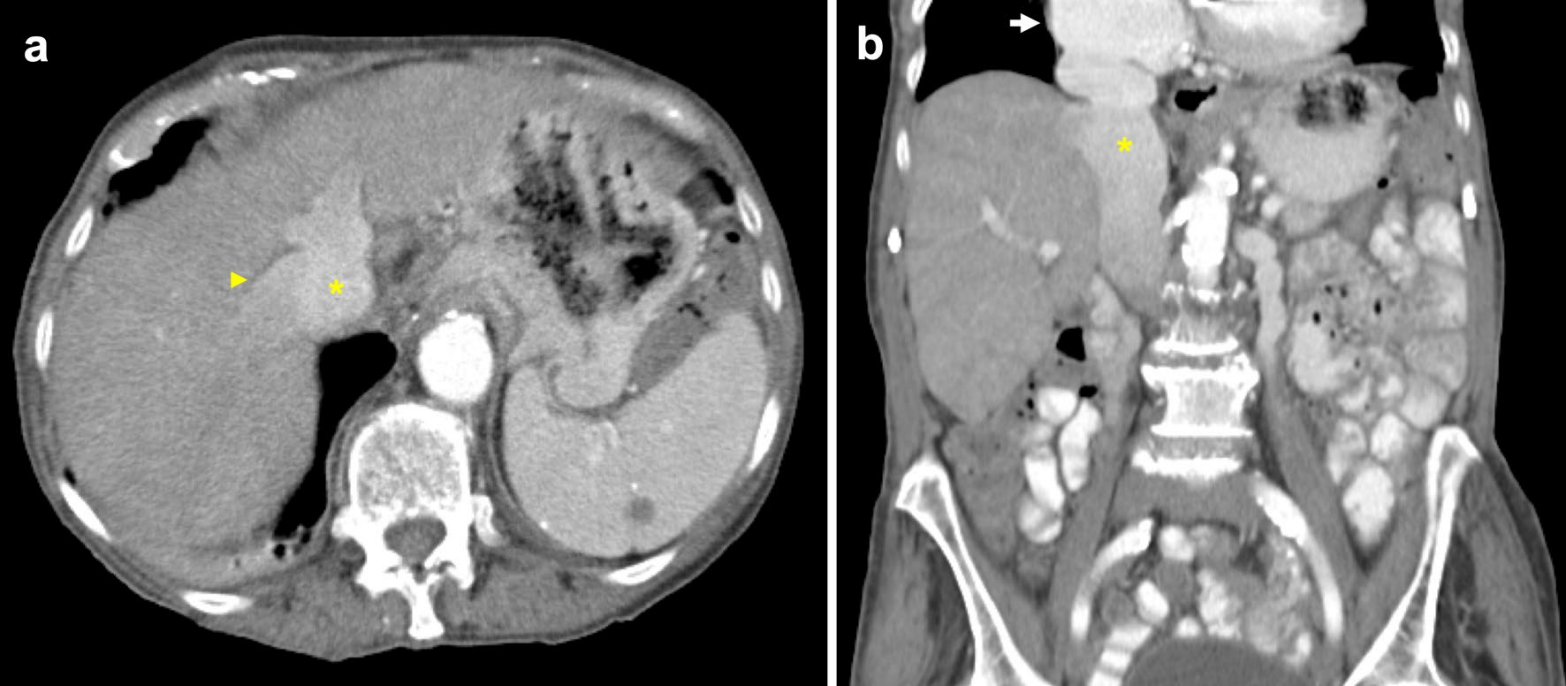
Dilated IVC from heart failure. a Axial and (b) coronal contrast-enhanced CT images demonstrating dilation of the IVC (asterisk) with hepatic reflux of contrast from the right atrium with early opacification (arrowhead) in a patient with clinically evident heart failure. The cardiomegaly (arrow) is partially imaged
